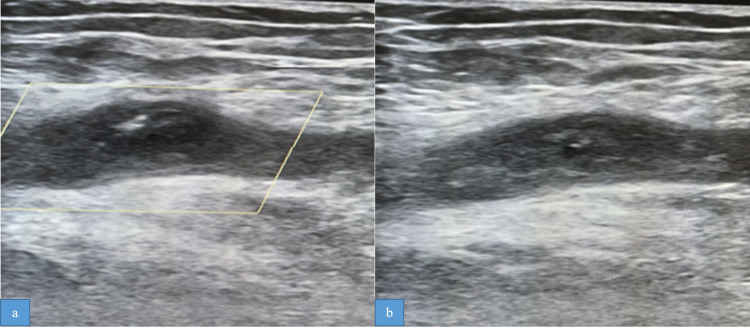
Doppler images. (a) Doppler study (gray scale) shows dilated non-compressible right common femoral vein with an echogenic intramural thrombus. (b) No color pick-up is seen on the color Doppler image.
